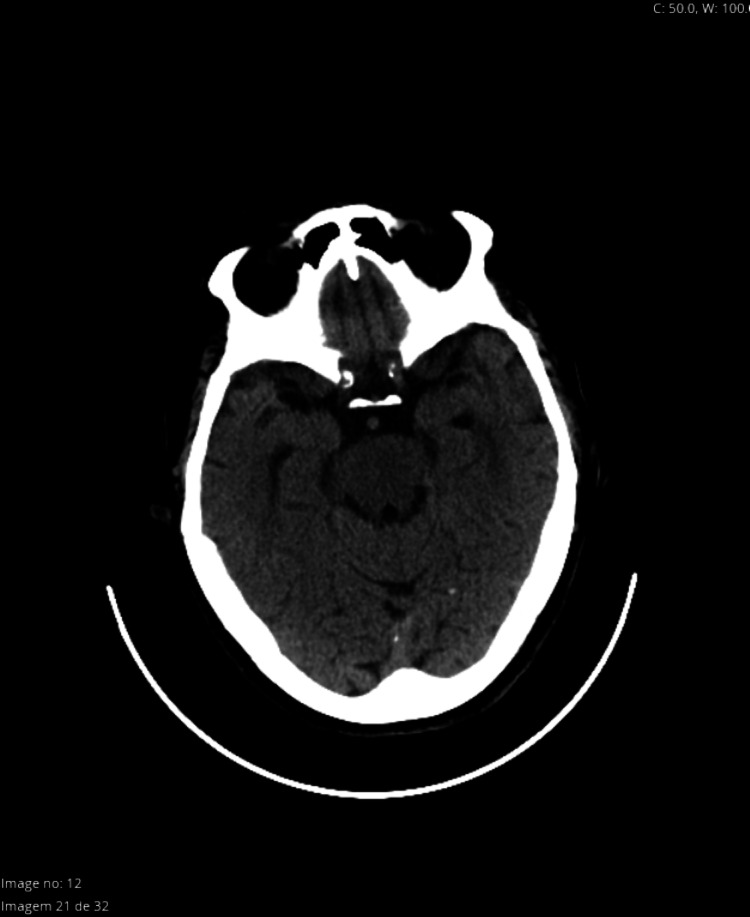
Cranioencephalic computed tomography appears normal
